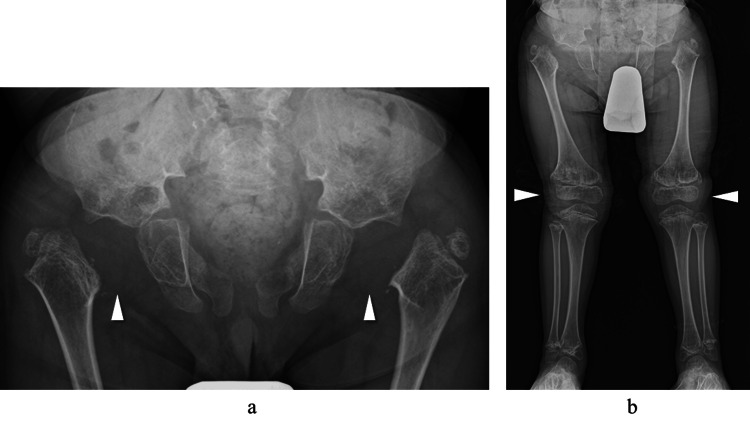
Plain radiographs at age 8. (a) Anteroposterior view of the hips: The femoral head is not visible, and lateral displacement of the proximal femur has progressed (white arrowheads). (b) Standing full-length view of the lower limbs: Bilateral genu valgum is observed (white arrowheads).
